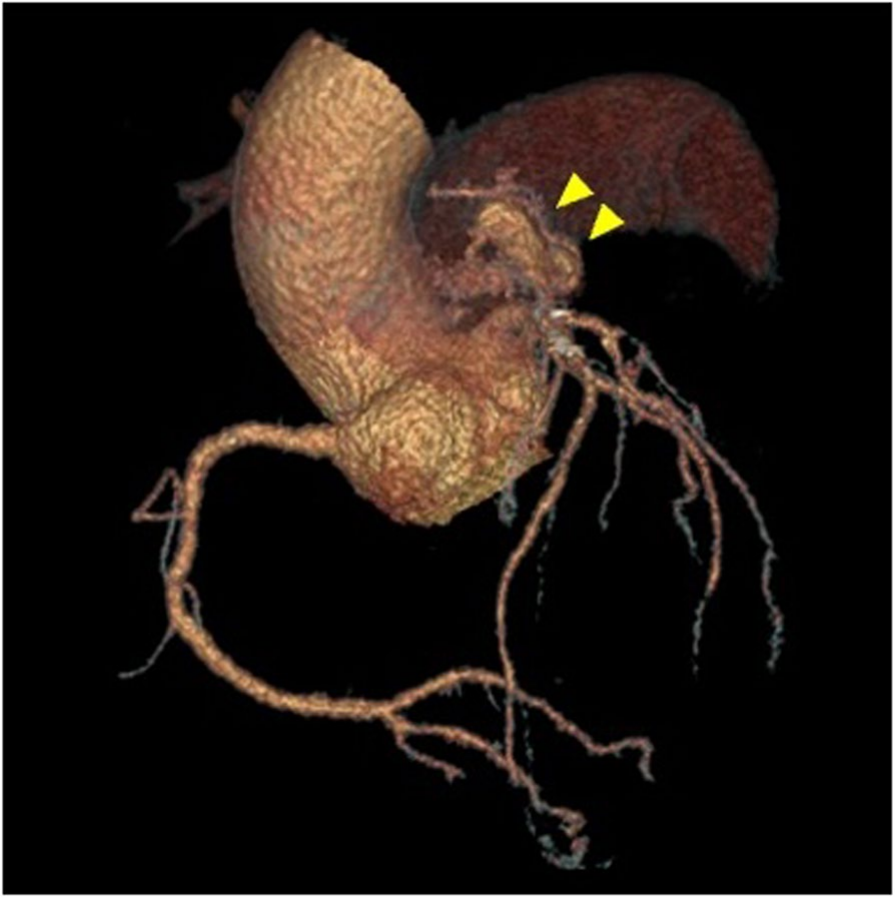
Preoperative coronary CT angiography of CAPAF. The fistula was tortuous with a partly dilatated tract from the left anterior descending artery into the main pulmonary artery (double arrowheads)
